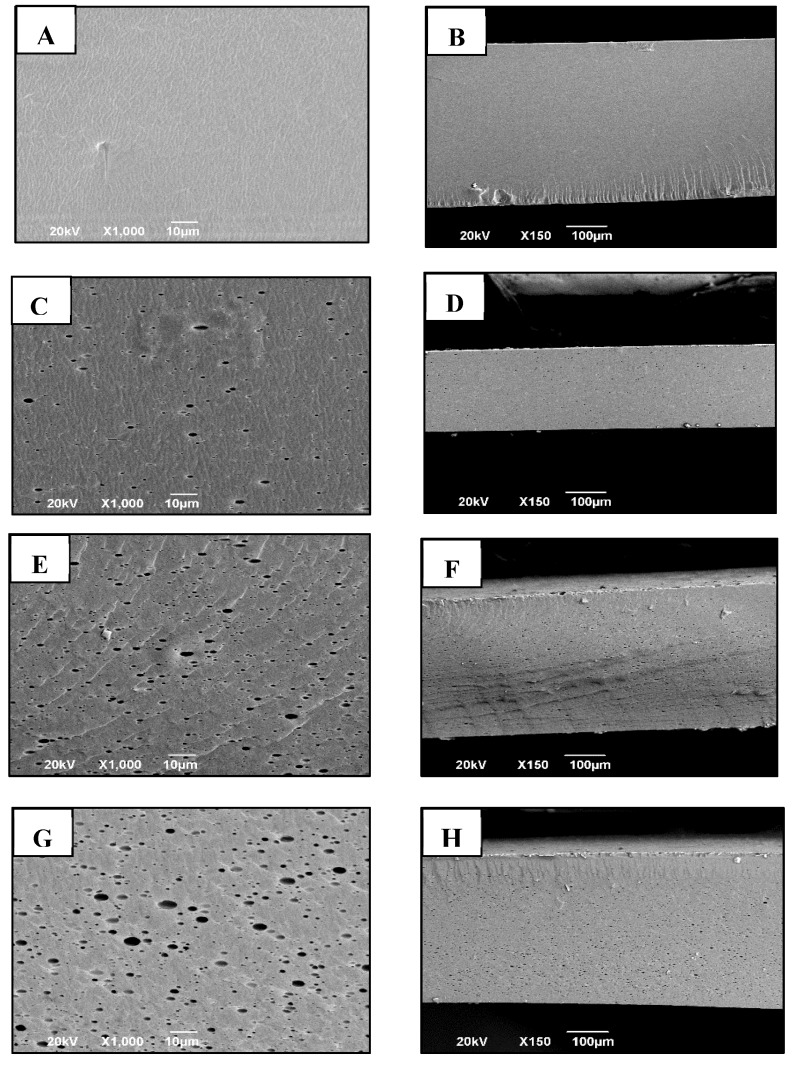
Cross-section analysis of CT-TCEO films with 0% (A) and (B), 0.5% (C) and (D), 1.0% (E) and (F), and 1.5% (G) and (H), at ×1000 and ×150 of magnification, respectively.
